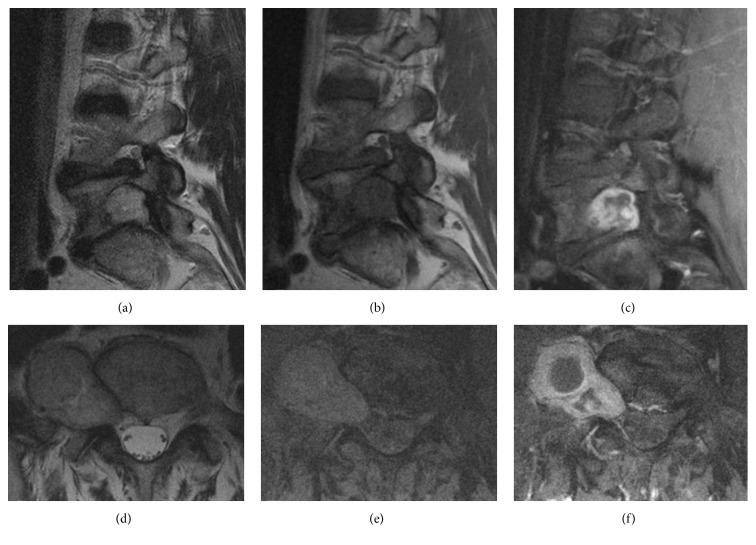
Preoperative spinal MRI. The lesion showed heterogeneous signal in T2WI (a, d), homogeneous low signal in T1WI (b, e, and f), and strong enhancement in postgadolinium examination (c, f). Displacement of the right psoas muscle can be noted (d, e, and f).
